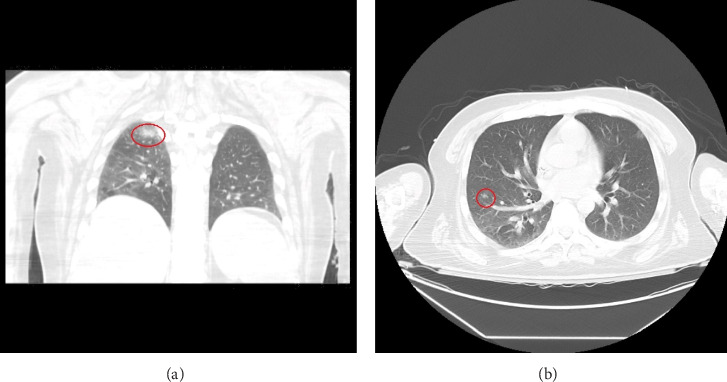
CT of the chest without IV contrast revealed a small area of subsegmental pneumonia in the superior segment of the right lower lobe ((a) encircle in red), along with multiple subcentimeter lung nodules ((b) the largest 6.1 mm nodule is encircled in red), accompanied by a small patch of ground-glass opacities in the right posterior lung base.
